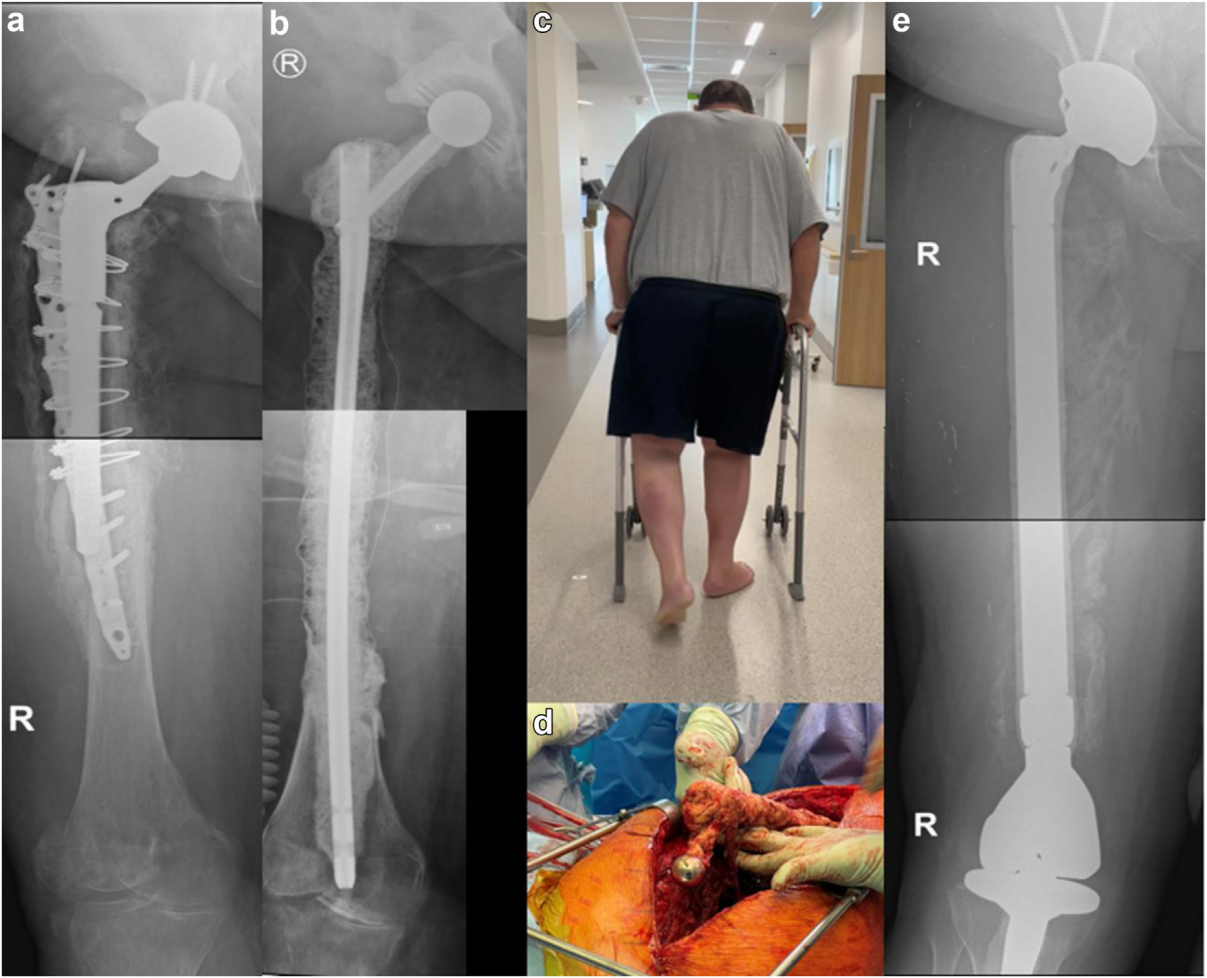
Case 2 RAH. (a) AP radiographs demonstrating the failed revision right THA with extensive osteomyelitis of the femur extending distal to the plate used to treat a periprosthetic fracture. (b) AP radiographs after the first-stage reconstruction of the femur using an antibiotic-cement-coated gamma nail as a temporary megaprosthesis. (c) The patient ambulating with a frame 1 week after his first-stage revision. (d) Clinical intraoperative images of interval prosthesis after hip dislocation at the second-stage revision. (e) AP radiographs of the reconstruction at the second-stage revision with a total femoral replacement prosthesis.
